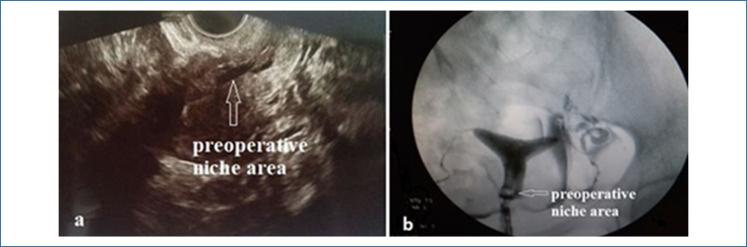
(a) Preoperative ultrasonographic image of the isthmocele niche, (b) Preoperative hysterosalpingographic image of the isthmocele niche.
